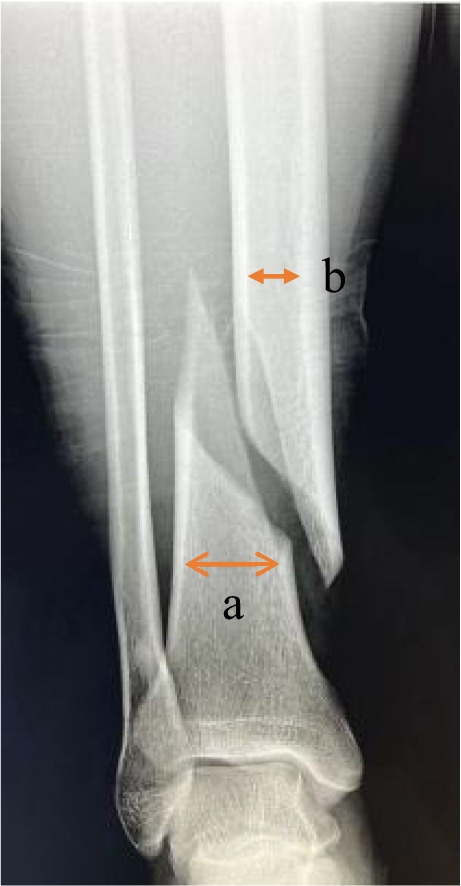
Anteroposterior radiograph. (a) The diameter of the fracture site was determined by the farthest intramedullary diameter of the fracture site; (b) diameter of the tibial isthmus; the “TFI ratio value” is defined as the ratio of the fracture site diameter to the intramedullary diameter of the tibial isthmus, which is a/b
